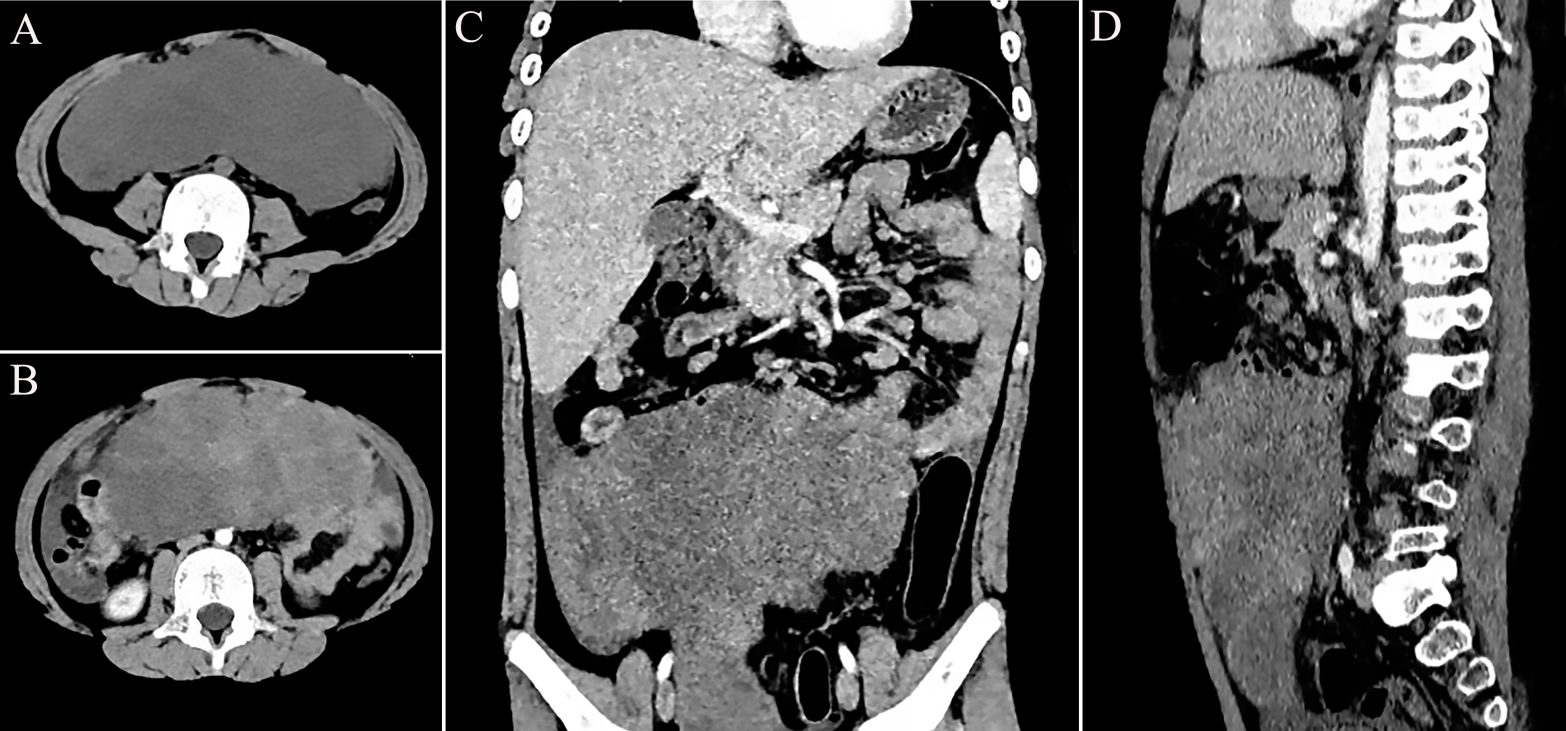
Preoperative imaging presentation of epithelioid inflammatory myofibroblastic sarcoma (EIMS) of the abdominal cavity. (A) Computed tomography (CT) of the horizontal position. (B) Enhanced CT of the horizontal position. (C) Enhanced CT of the coronal position. (D) Enhanced CT of the sagittal position.
